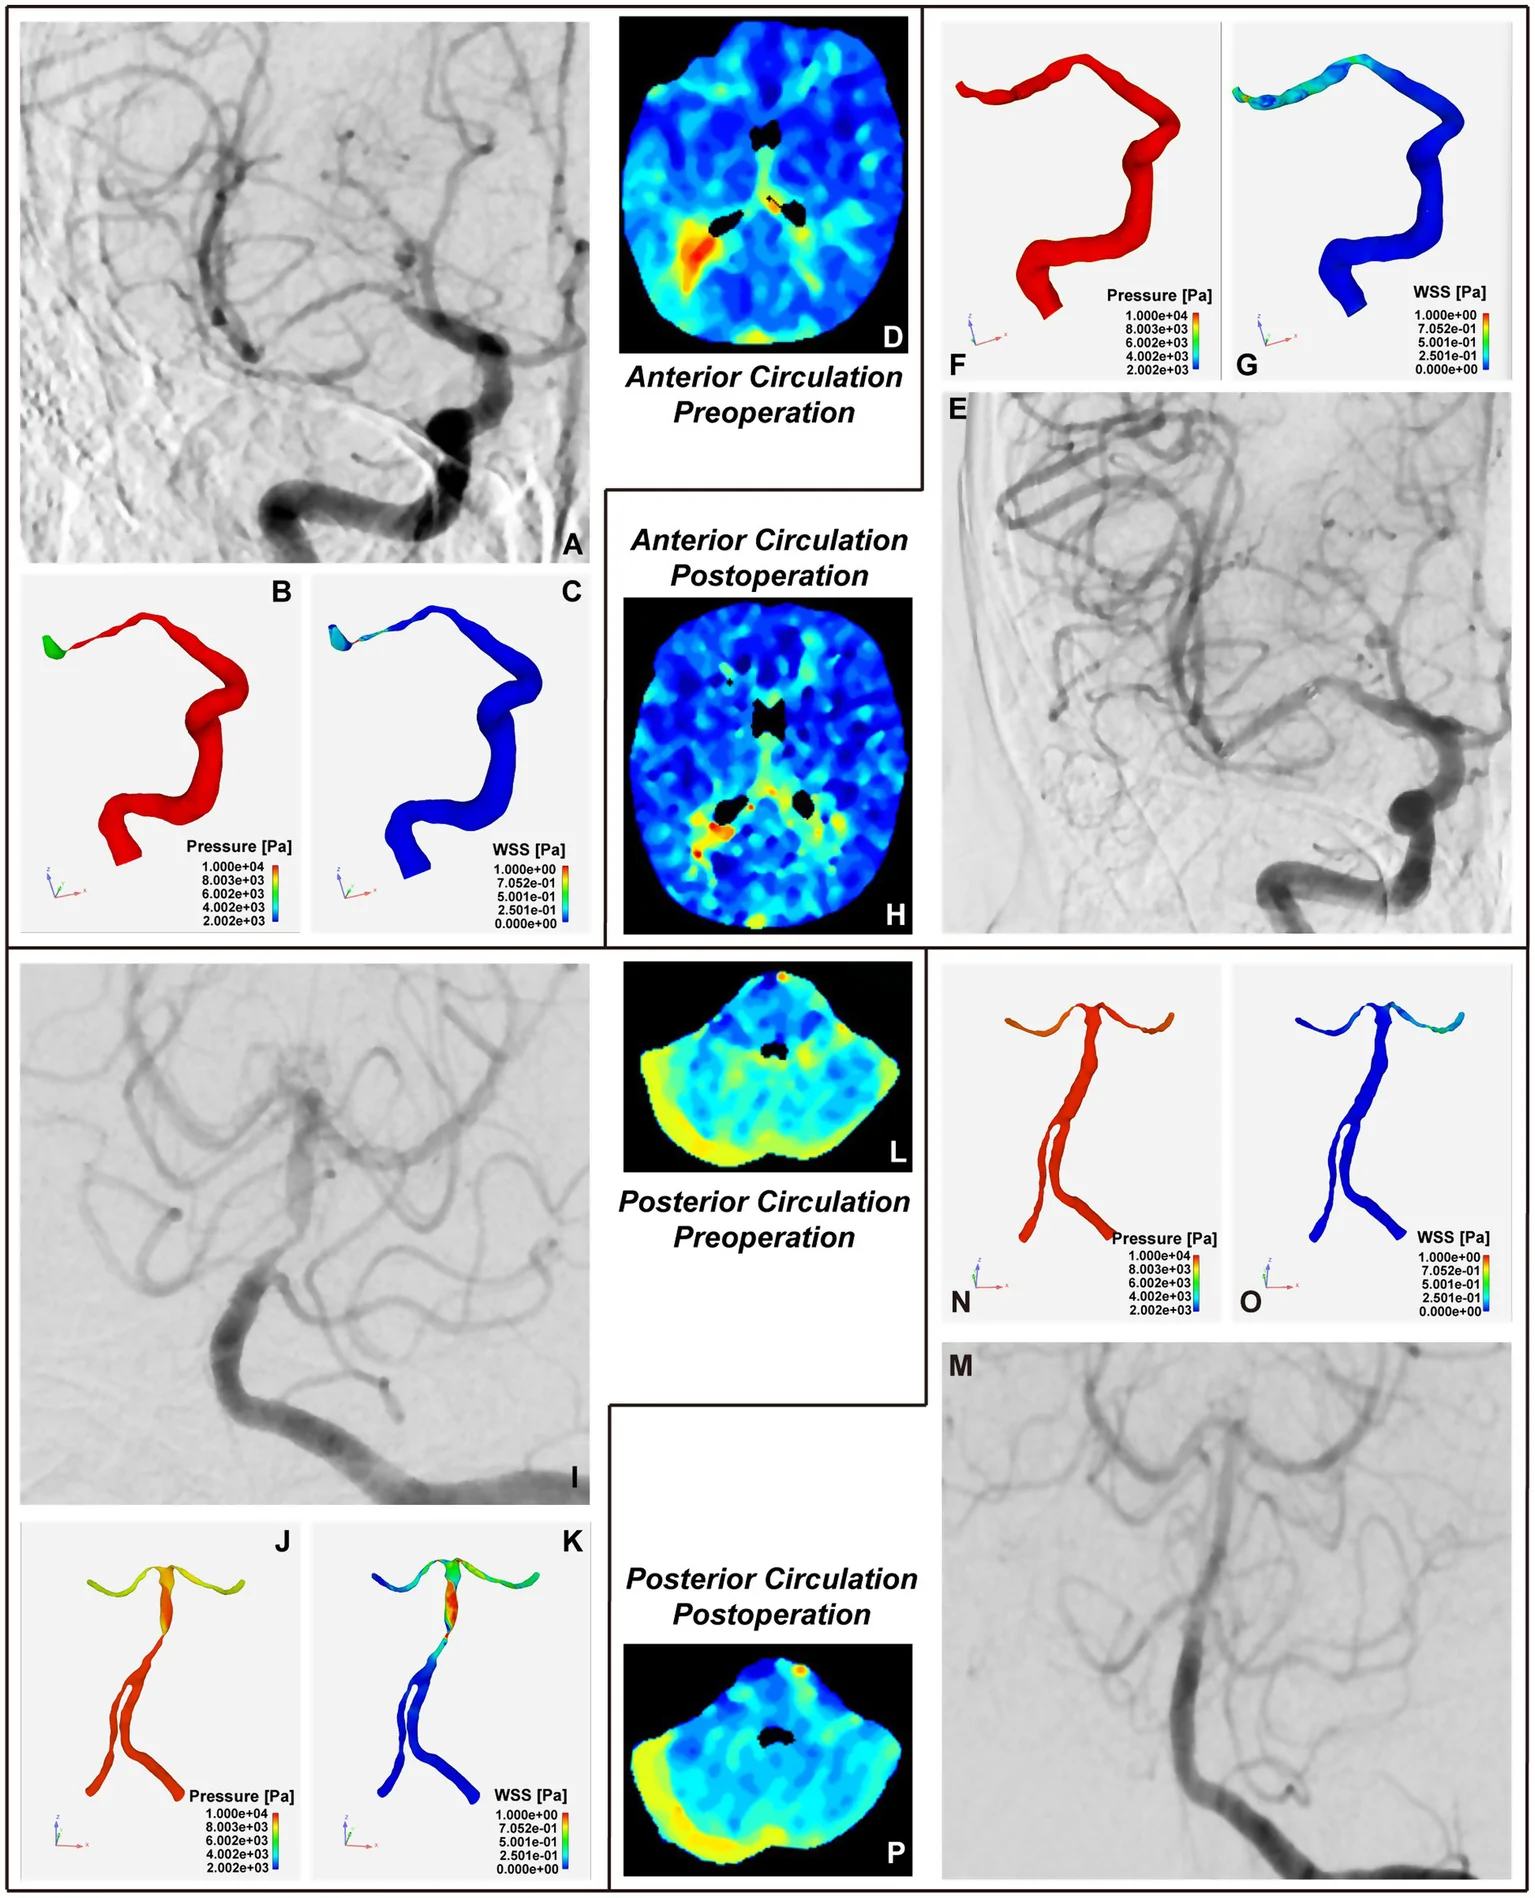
Representative multimodal images before and after intracranial artery stenting in anterior and posterior circulation lesions. Anterior circulation case: before stenting, (A) DSA image showing severe right middle cerebral artery stenosis; (B) CFD-derived pressure ratio map; (C) CFD-derived wall shear stress ratio map; and (D) CT perfusion map. After stenting, (E) DSA image showing improvement of right middle cerebral artery stenosis; (F) increased CFD-derived pressure ratio; (G) reduced wall shear stress ratio; and (H) CT perfusion map showing improvement in perfusion-delay abnormalities. Posterior circulation case: before stenting, (I) DSA image showing severe basilar artery stenosis; (J) CFD-derived pressure ratio map; (K) CFD-derived wall shear stress ratio map; and (L) CT perfusion map. After stenting, (M) DSA image showing improvement of basilar artery stenosis; (N) increased CFD-derived pressure ratio; (O) reduced wall shear stress ratio; and (P) CT perfusion map showing improvement in perfusion-delay abnormalities.
